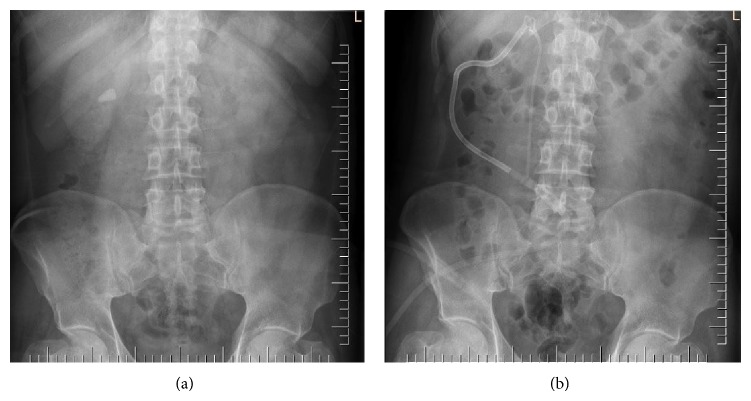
Preoperative (a) and postoperative (b) X-rays of a patient with a 21 mm diameter kidney stone treated via percutaneous nephrolithotomy.
